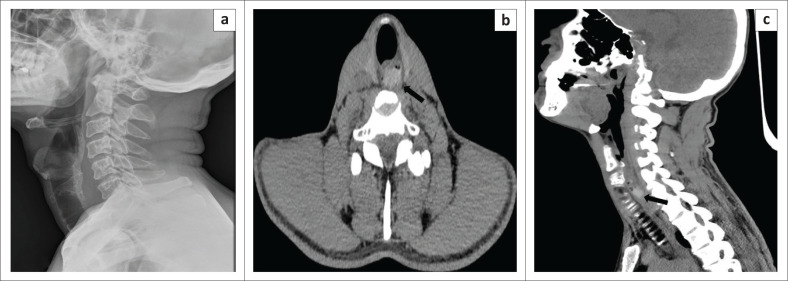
Food bolus (meat) impaction in a 70-year-old male. (a) Lateral radiograph of the neck was unremarkable. Computed tomography (b) axial and (c) sagittal reformatted images shows the impacted bolus of food (black arrow) in the hypopharynx at the C6–C7 level.
